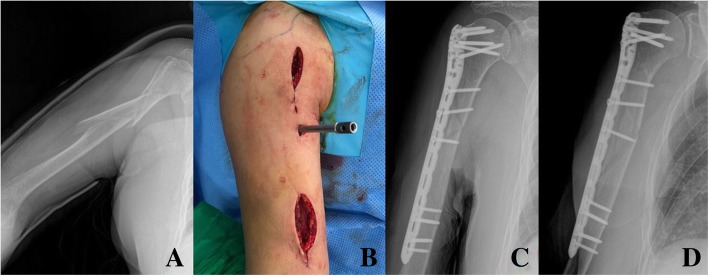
In a 85 year-old female patients after slip down injury, X-ray showed long spiral (Complex spiral type, AO classification: C1) humerus shaft fracture with proximal fracture extension to the greater tuberosity (a). In the midportion of the long philos plate, additional locking or compression screws were inserted through several small incisions for further rigid fixation (b). Long humeral philos plate could fix this complex humeral shaft fracture adequately (c, d)
